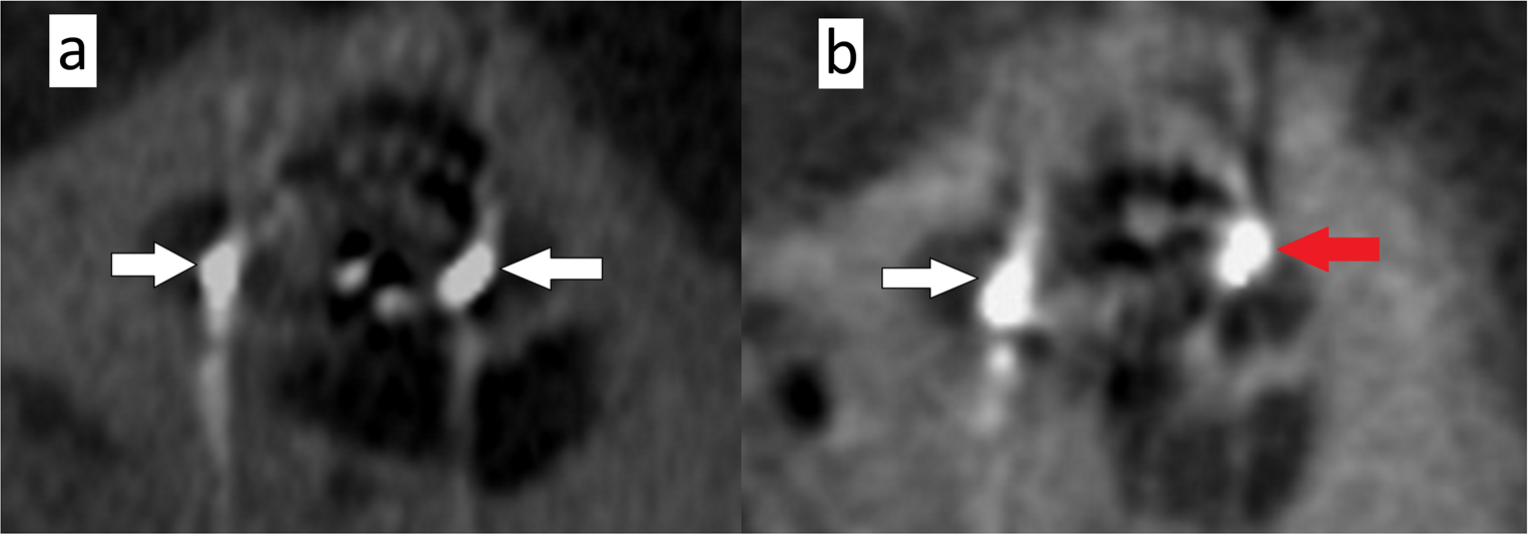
Midmodiolar view reformats of CBCT images: a shows the scala tympani insertion of the electrode at the basal turn of the cochlea ( white arrows); b shows the shift of the electrode from scala tympani (white arrow) to scala vestibuli (red arrow) inside the cochlea*Parasagittal view for the facial recess*: oblique sagittal view obtained in a plane parallel to the superior semicircular canal [11] (Fig. 5):
